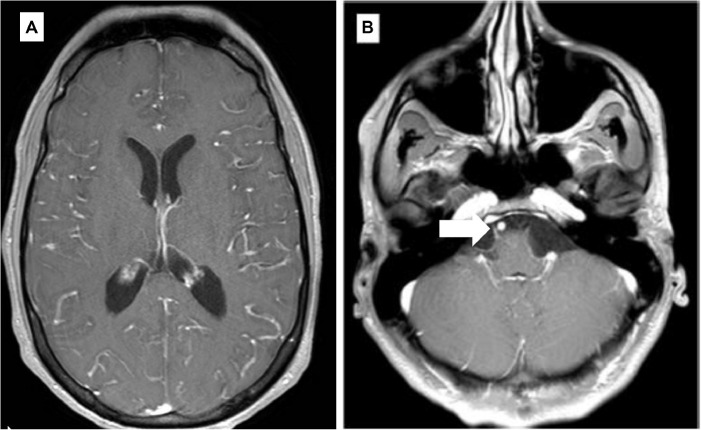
Selected axial T1 weighted images from brain MRI with contrast (A,B). (A) Diffuse gyriform leptomeningeal enhancement on both cerebral hemispheres. (B) Enhancement of the cisternal portion of the right 6th cranial nerve (arrow).
